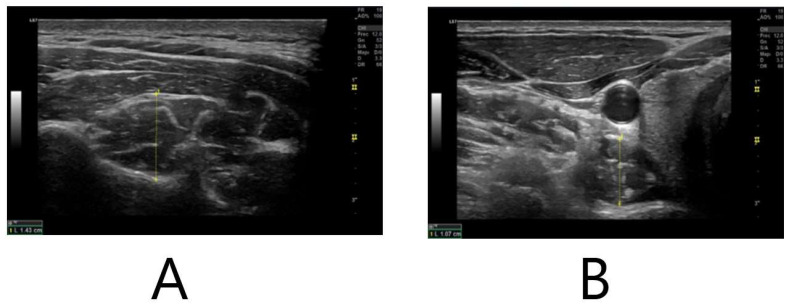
Ultrasound assessment of deep cervical muscles. (A) Ultrasound image of the multifidus in transverse view at C5. The caliper is placed 90⁰ to the lamina of C5 where the rater considered the muscle to be at its thickest and up to the echogenic line of the hyperechoic fascia between the semispinalis cervicis and semispinalis capitis. (B) Ultrasound image of the longus colli at C6 transverse view. The caliper is placed on the midpoint of the ventral surface of the C6 vertebral body and the interface between the Lcol and the pre-fascial tissue surrounding the carotid artery.
